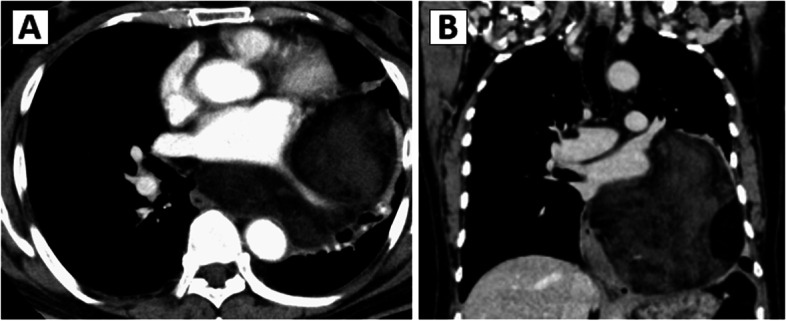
The chest computed tomography image showed a posterior mediastinal tumor measuring 160 × 140 × 120 mm displacing and invading the left lung lower lobe and esophagus
